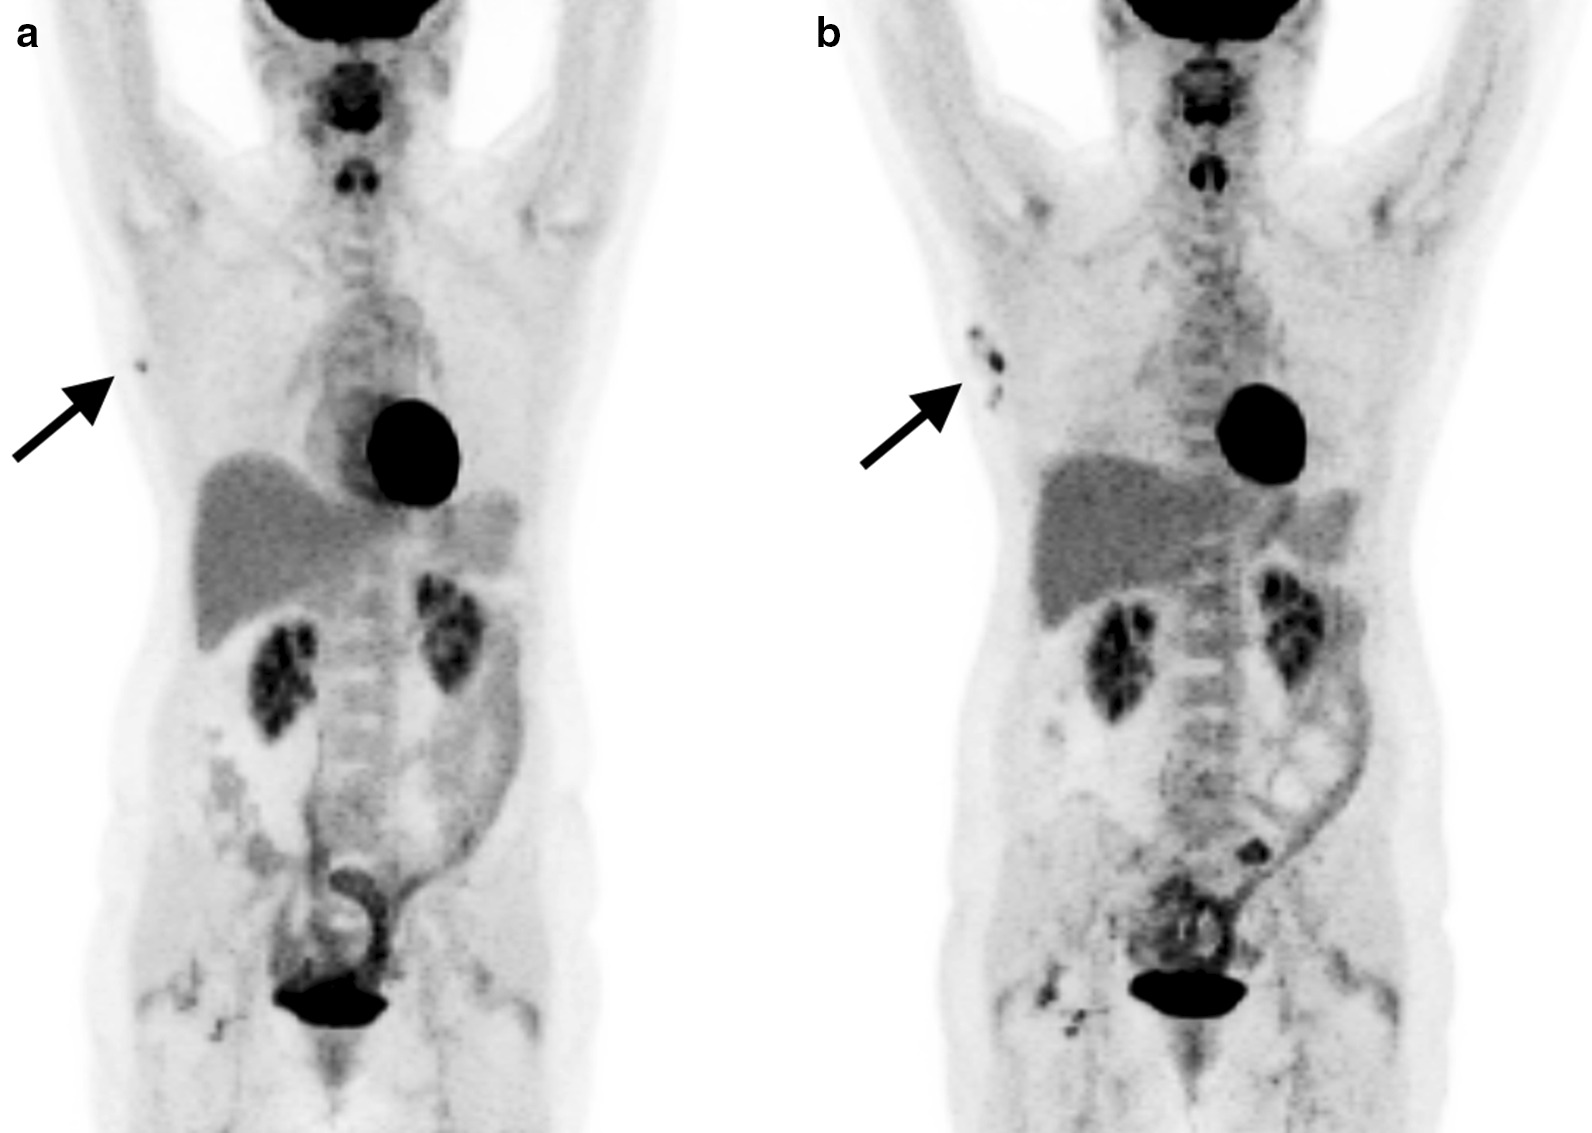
a PET maximum intensity projection (MIP) image of patient with right axillary node metastasis at baseline with SUL_peak_ of 1.62 and tumor volume of less than 1.00 cc. Though visually perceptible, Auto-PERCIST™ failed to detect the lesion due to small size. b On the follow-up MIP image, the number of metastatic nodes and the [^18^F]FDG uptake intensity are increased to SUL_peak_ of 2.84, allowing detection by Auto-PERCIST™
